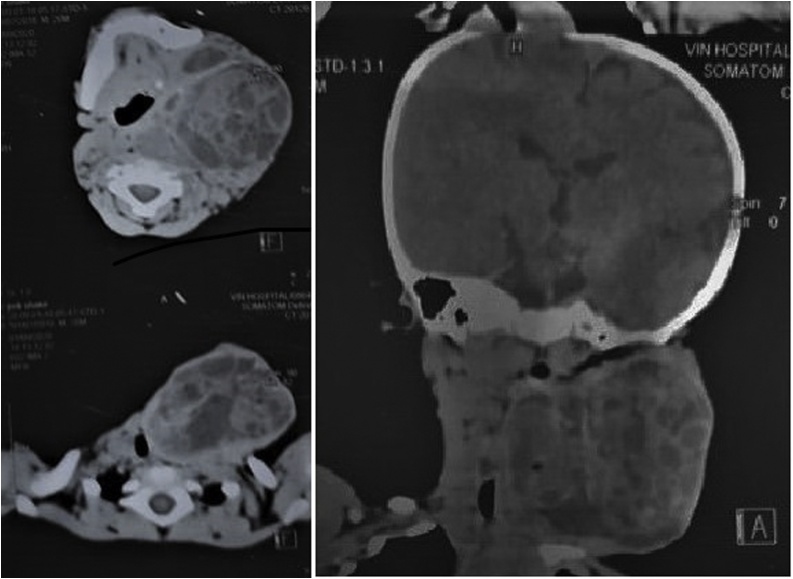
CT-scan of the head and neck showing an evidence of enhancing multi-cystic lesion measuring 10 × 8 × 8 cm with multiple flecks of calcification. The mass is extending to the mastoid bone and the clavicle and causing displacement of the trachea, esophagus, and carotid sheath to the opposite side.
